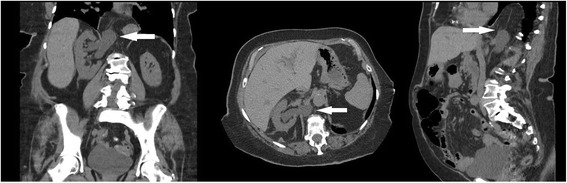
Follow up computed tomography (CT) 18 months later demonstrating increased hydroureteronephrosis and increased segment of entrapped ureter. Coronal, Axial, Sagittal views with white arrow showing segment of herniated ureter
